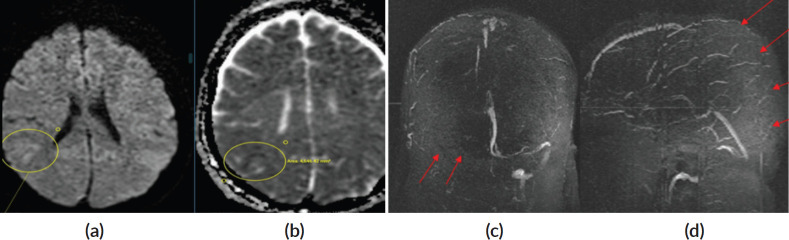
(a and b): Diffusion-weighted imaging (DWI) and Apparent Diffusion coefficient (ADC) images showing diffusion restriction with corresponding low ADC (marked with circle) in right parietal lobe suggesting acute infarction. (c): Non-contrast 3D time of flight (TOF) MR venogram depicts non-visualisation of right transverse and sigmoid sinus – Suggestive of complete thrombosis (red arrows). (d): Non-contrast 3D TOF MR venogram depicts non-visualisation of posterior two third superior sagittal sinus – Suggestive of complete thrombosis (red arrows).
